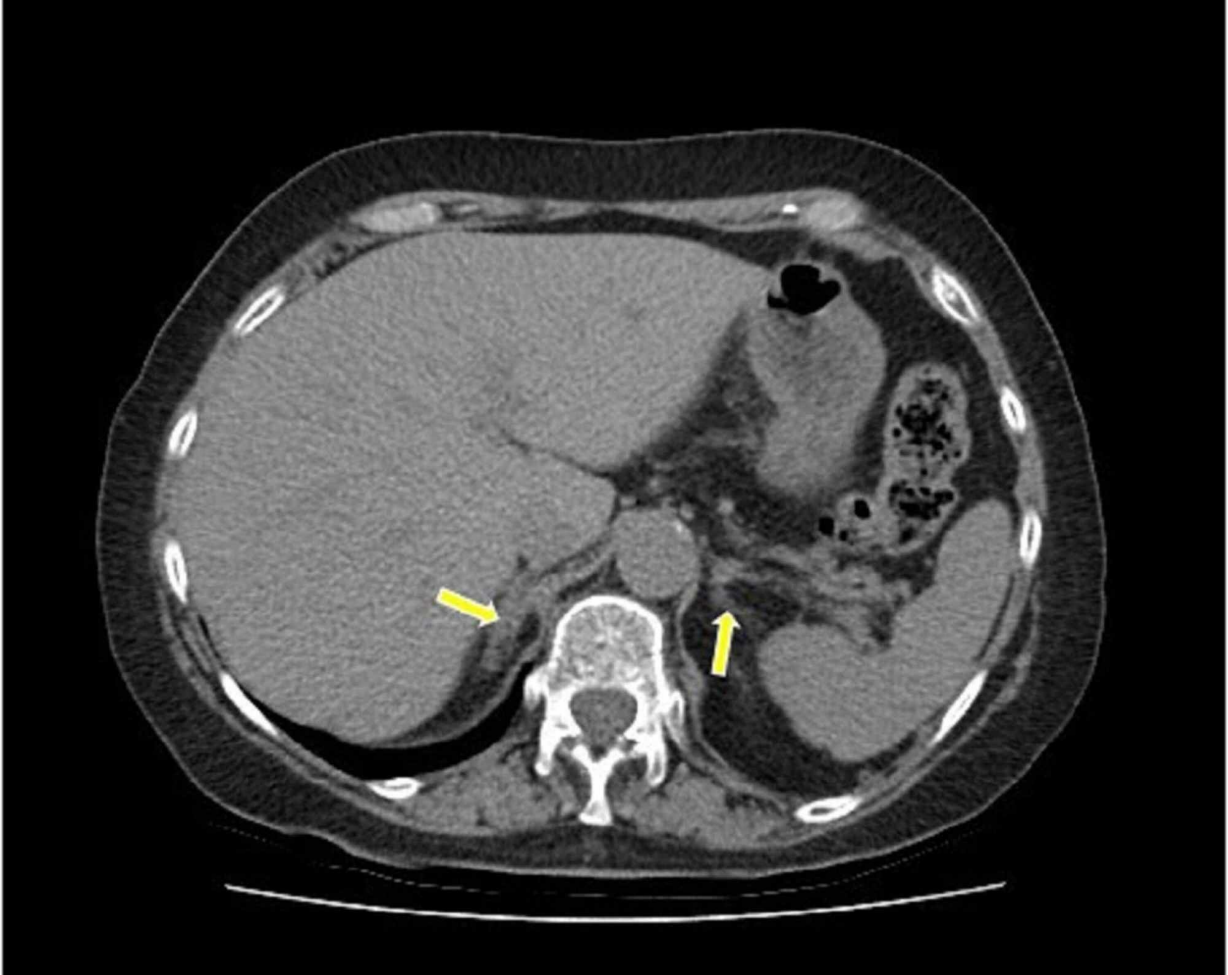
Follow-up CT scan demonstrating resolution of the bilateral adrenal hematomas
